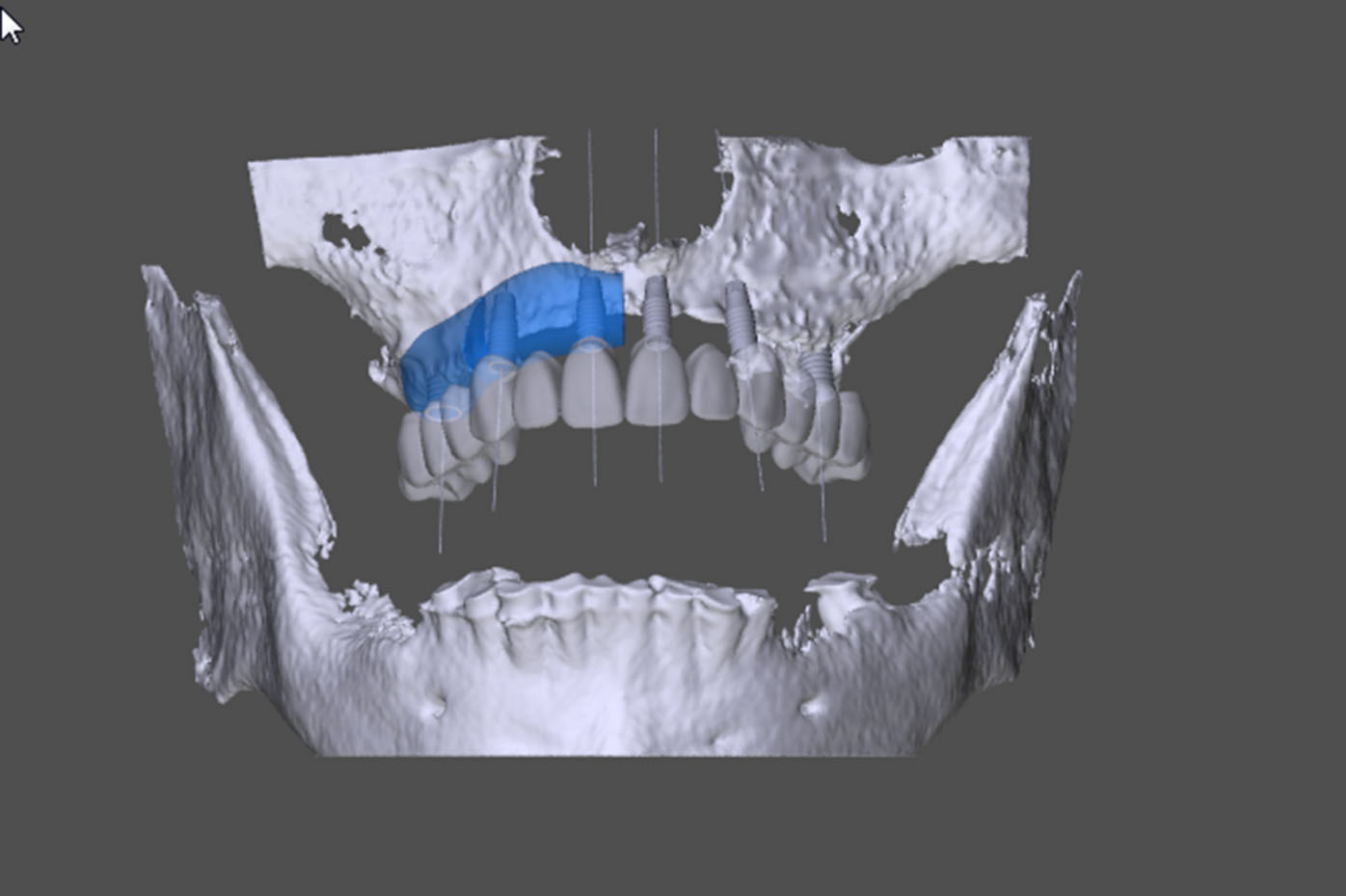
A virtual model of a CBCT for advanced backward planning of prosthetics and dental implants (grey) and customized milled Bonebuilder® for the maxilla (Blue; Botiss®-Software; Botiss-Straumann, CH-Basel). Anterior view
